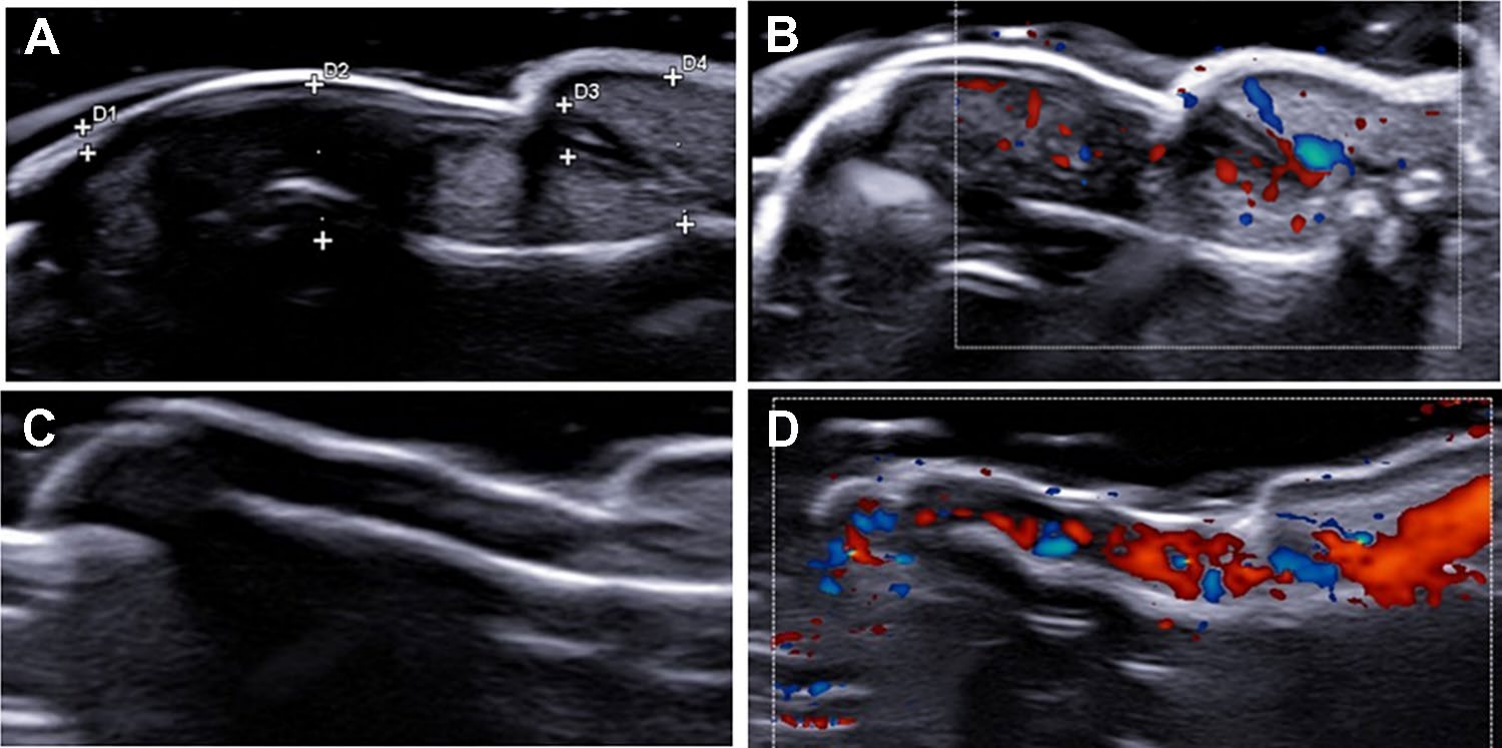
HFUS nail evaluation. PATIENT 1: A structural nail changes characterized by inhomogeneous echogenicity nail bed (d1), enlarged nail enthesis (d2), thickened matrix (d4) and plate (d3) with overall increased blood flow (B); PATIENT 2: C normal nail morphology without and with an increased Doppler signal (D)
